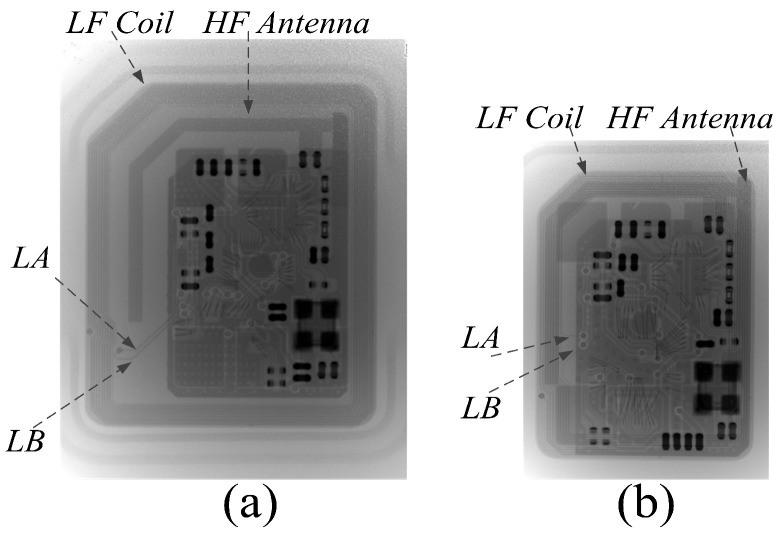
X-ray of SIM cards. (a) Micro-card. (b) Nano-card.
